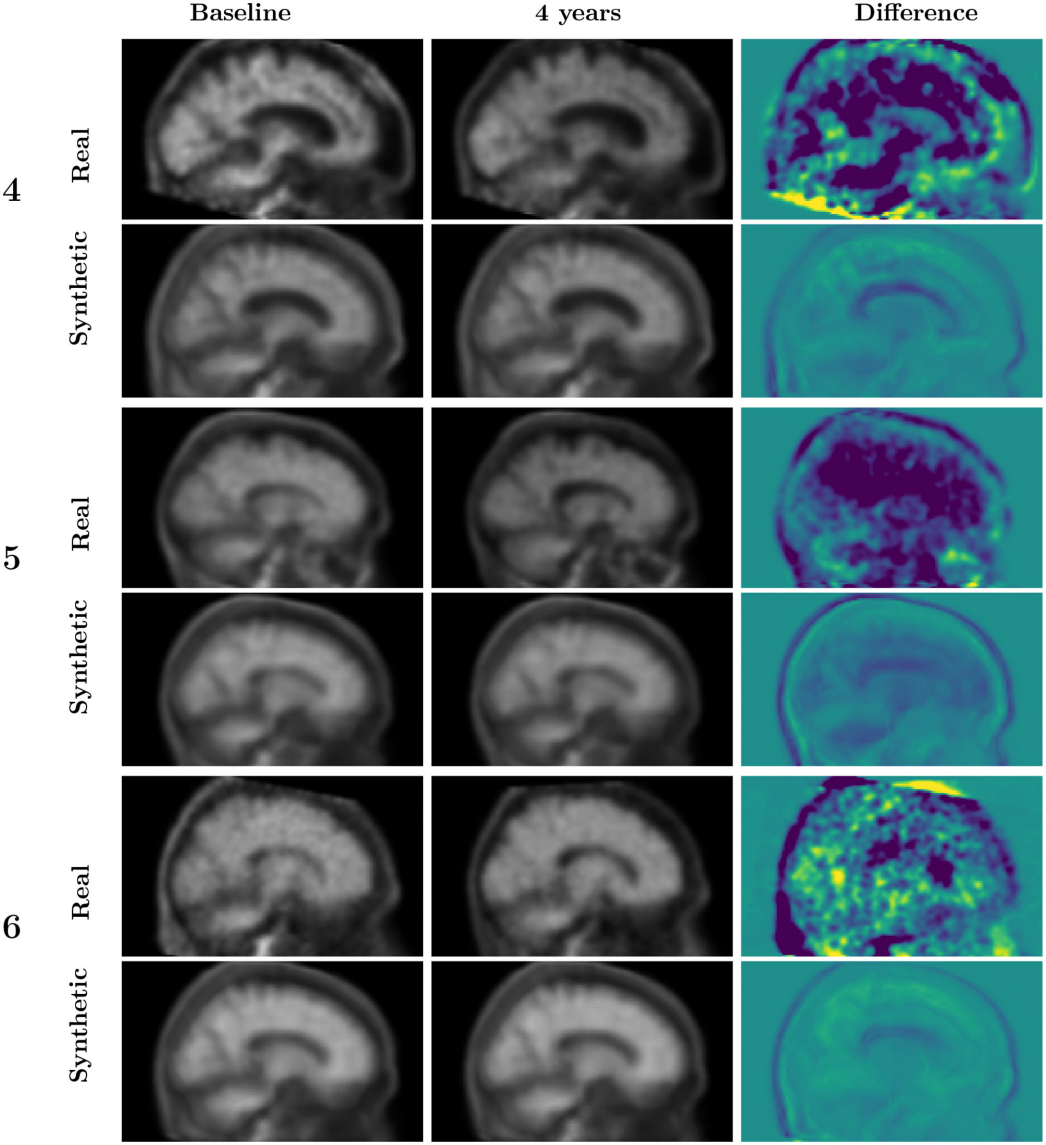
Synthetic vs. observed four-year progression for amyloid-positive and stable participants, i.e., those without significant brain amyloid accumulation as measured by SUVR change. See Figure 4 for the corresponding latent representation.
